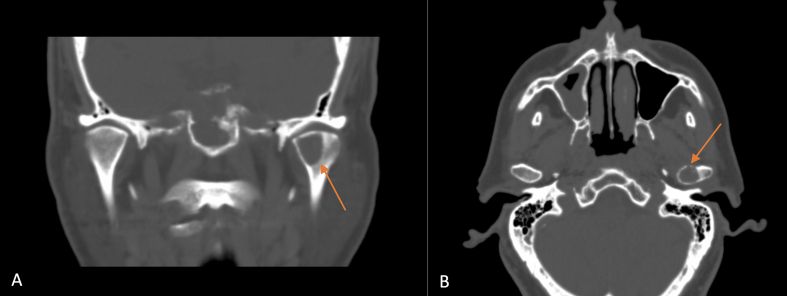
Coronal (A) and Axial (B) CT images of osteolytic lesion in the left mandibular condyle.
